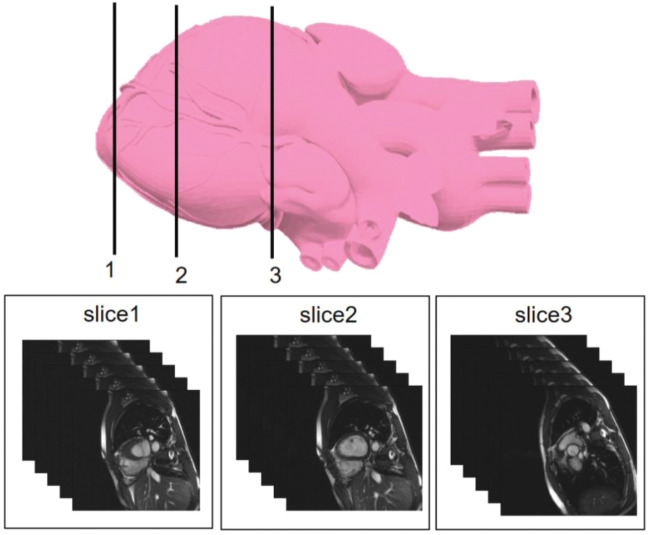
Cardiac MRI sampling locations included in this study.
